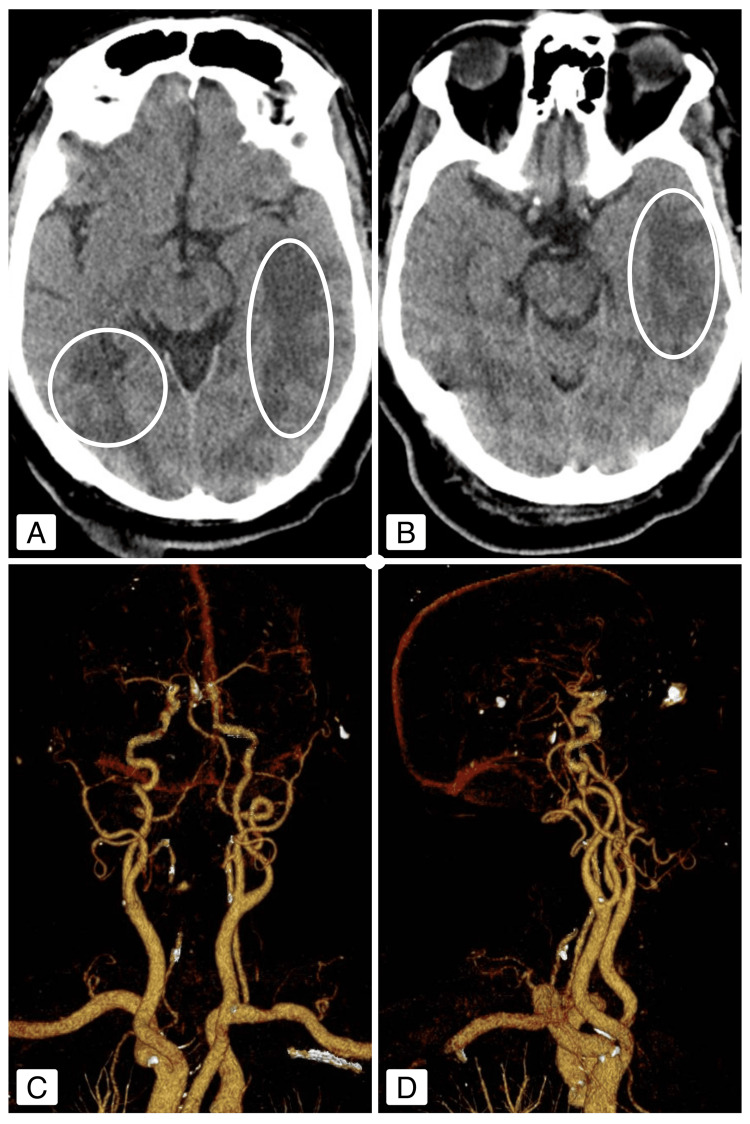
(A-B) Brain computed tomography (CT) showing bilateral hypodense areas in the temporo-occipital regions, more extensive on the left, with additional involvement of the anterior temporal and parietal lobes (circles highlighting the areas of lesion). (C-D) Computed tomography angiography (CTA) of the cervical and intracranial vessels with three-dimensional (3D) reconstruction, showing no significant stenosis or occlusion of the visualized arteries.
